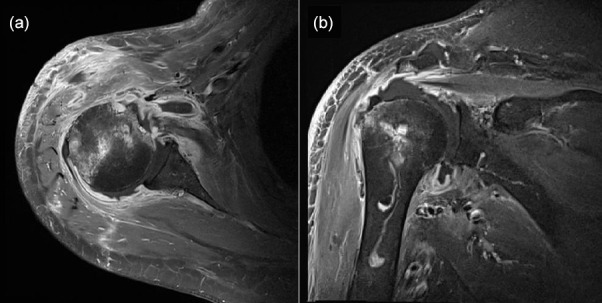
Enhanced magnetic resonance images showing effusion in the right glenohumeral joint with synovial hypertrophy and combined diffuse heterogeneous enhancement along the right glenohumeral joint capsule. Additionally, an ill-defined bone marrow signal intensity change is observed in the right humeral head. Panel (a) presents a T1-weighted, contrast-enhanced fat suppression axial-view image, whereas panel (b) shows a T1-weighted, contrast-enhanced fat suppression coronal-view image.
